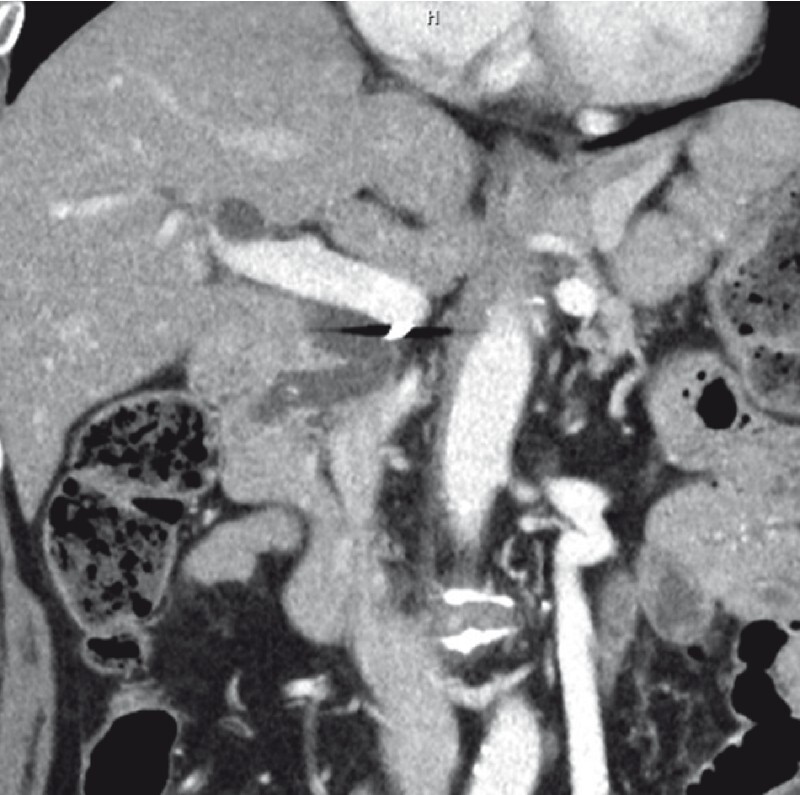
Computed tomography (CT) revealed a stone in the tortuous bile duct.
